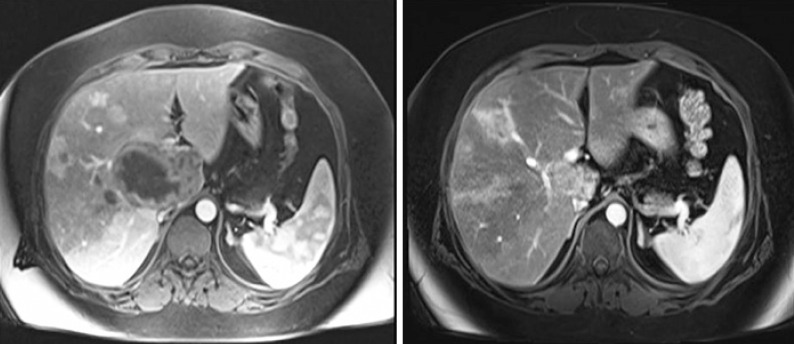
Magnetic resonance imaging before embolization and one year later. Hypervascular lesions in the liver right lobe. The largest one is located in the caudate lobe with central necrosis and external compression of the inferior vena cava. The control magnetic resonance imaging exam performed one year after embolization shows a reduced hypervascular lesion in the caudate lobe, no external compression of the inferior vena cava, and no growth of the remaining hypervascular lesions
